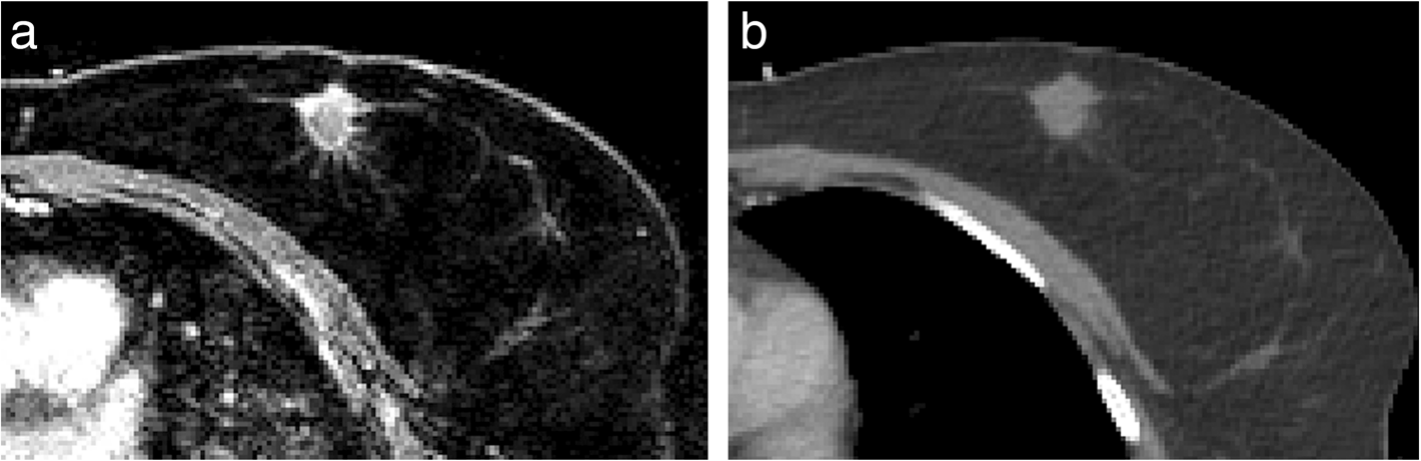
Small peripheral branches in the transversal plane. (a) CE-MRI and (b) CE-CT.
